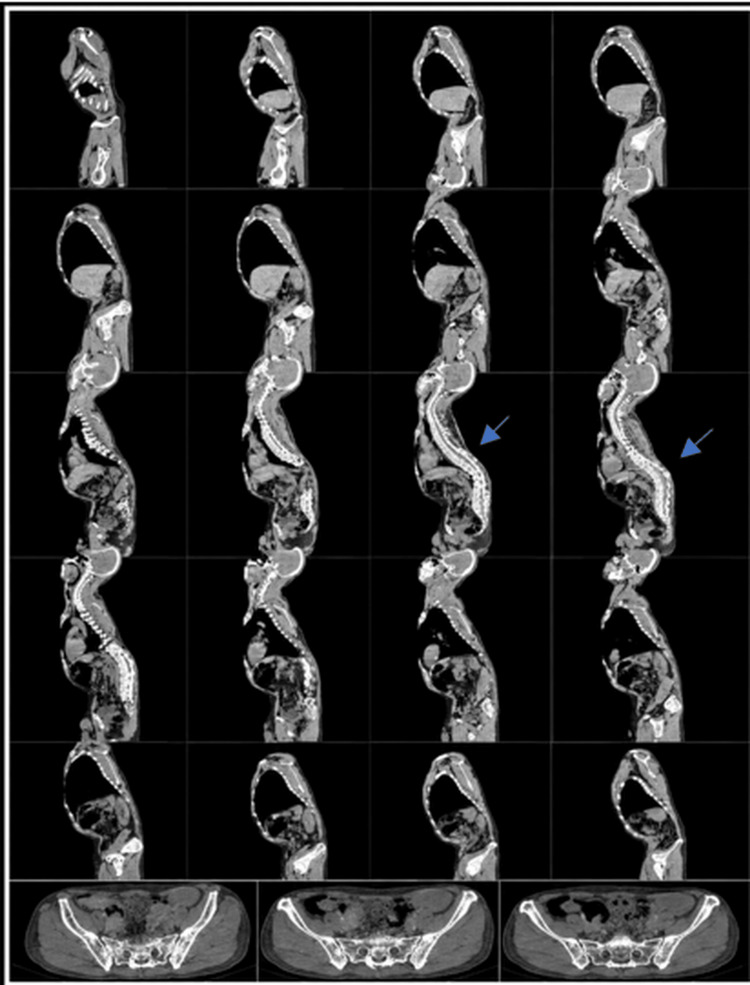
Whole-spine sagittal MRI images and axial pelvic MRI sections demonstrating advanced ankylosing spondylitis with extensive syndesmophyte formation, bamboo spine appearance, and fusion of the posterior vertebral elements (arrows).
